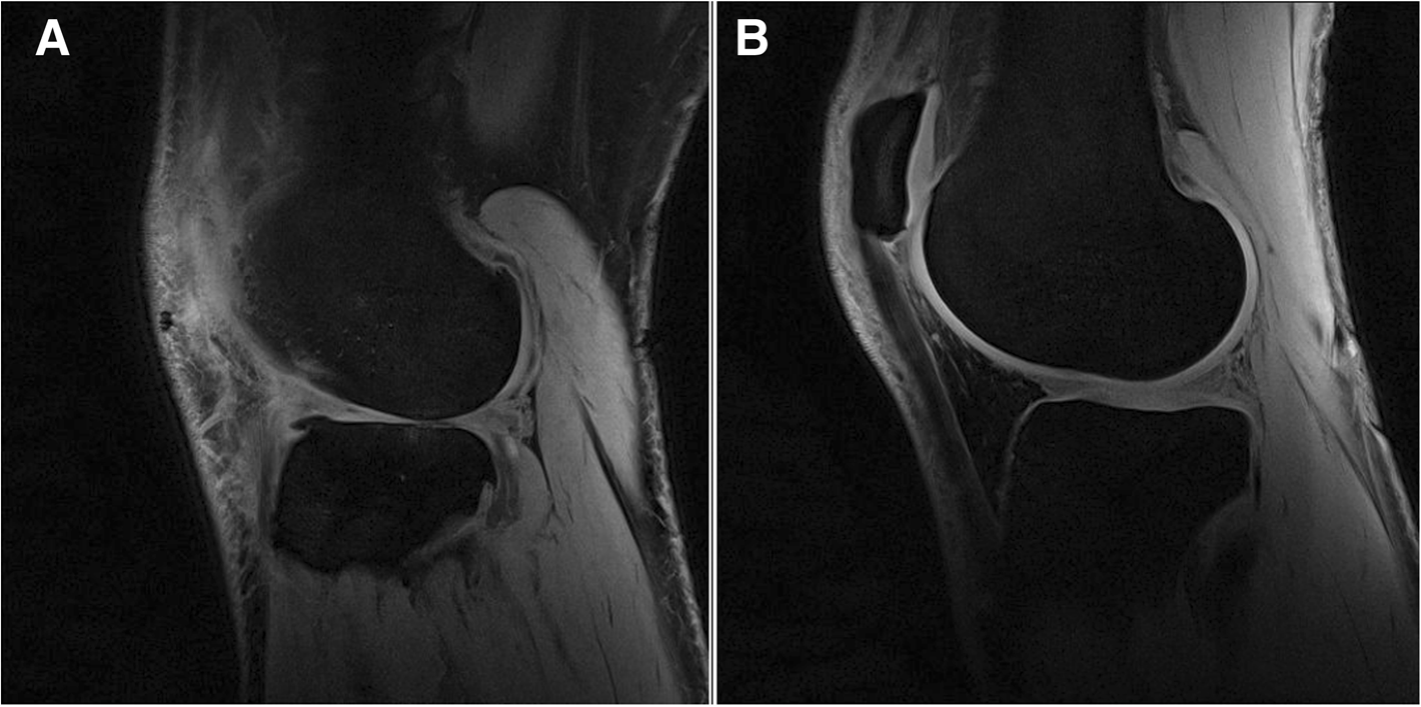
Lateral compartment of male participants with a full thickness cartilage damage of the lateral femur and tibia and b) without cartilage lesions depicted using sagittal 3D VIBE MRI
